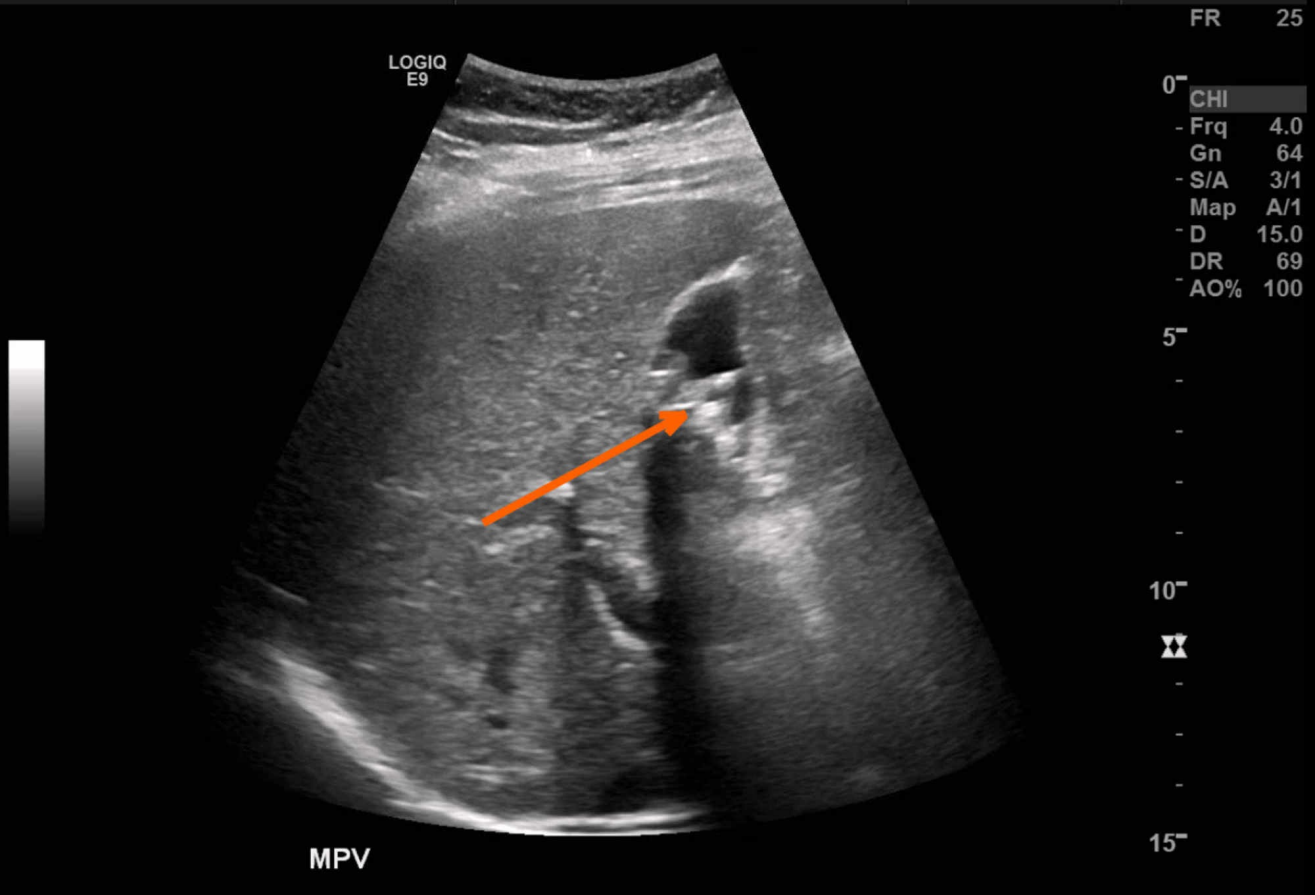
Abdominal ultrasound of the right upper quadrant No pericystic fluid or gallbladder wall thickening. The arrow pointing to impacted stone in gallbladder neck.
